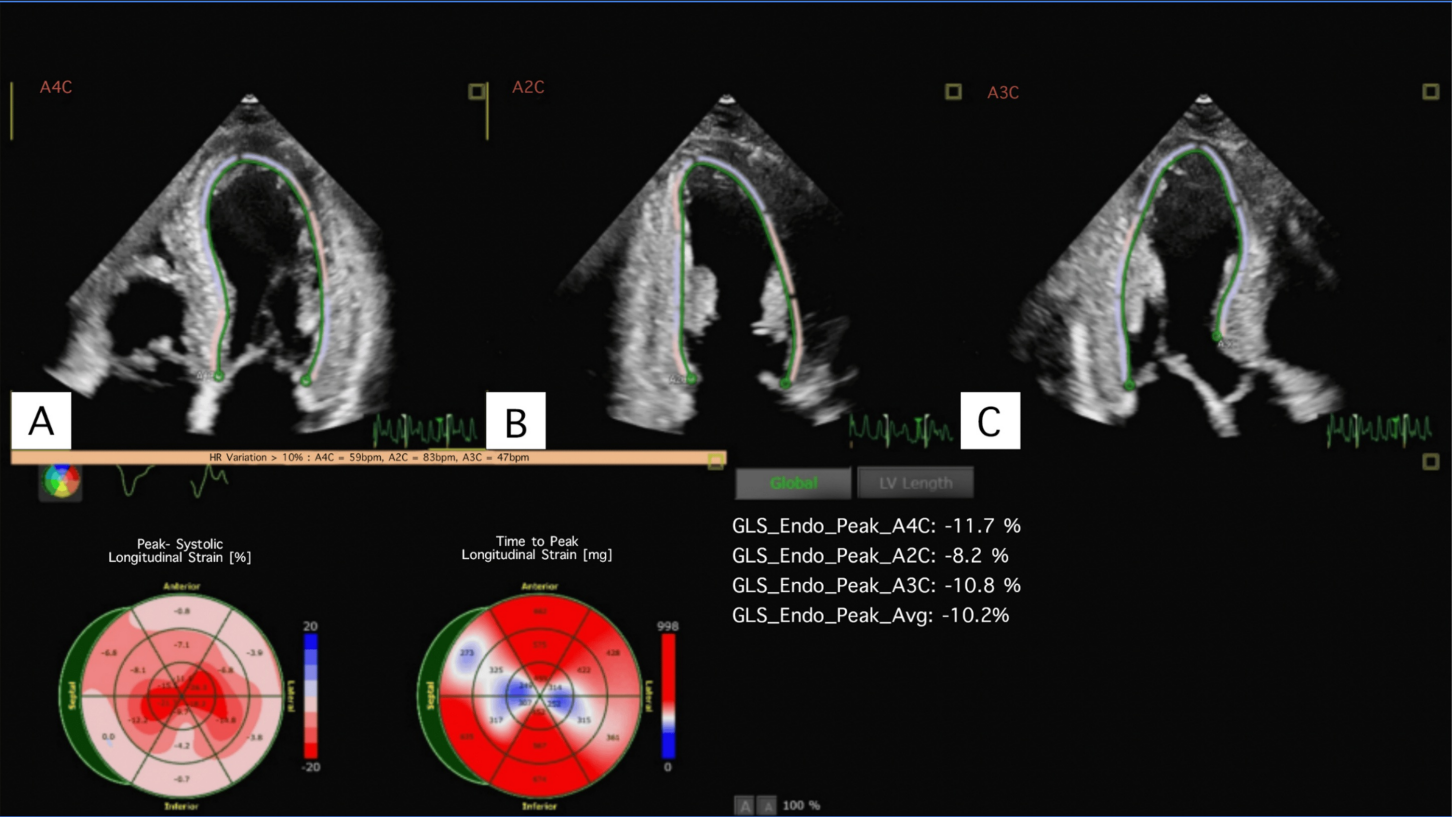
Classic strain pattern demonstrated in panel A (apical 4-chamber), panel B (apical 2-chamber), and panel C (apical 3-chamber). The pattern, associated with cardiac amyloidosis, shows reduced overall global longitudinal strain with relative apical sparing, referred to as the “cherry on top” sign
